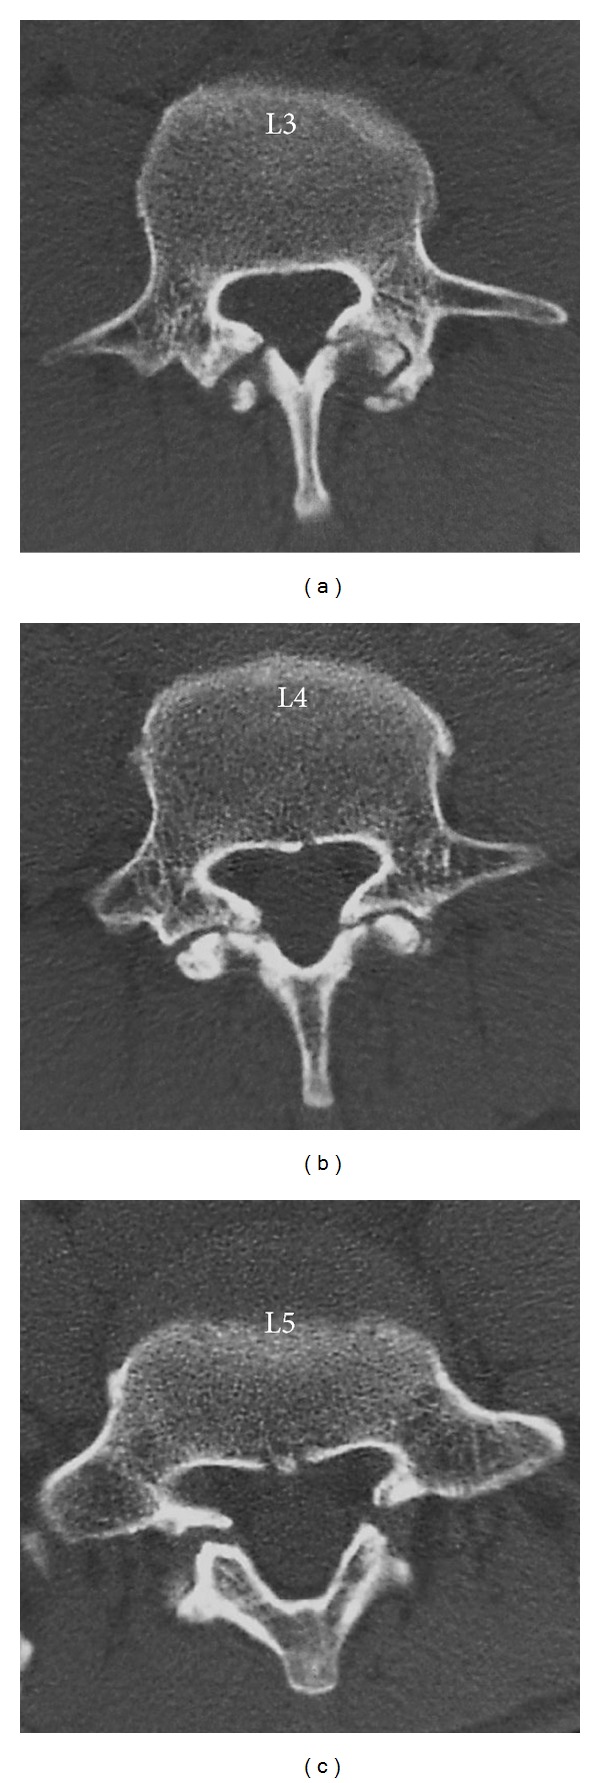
CT at initial presentation shows pseudarthrosis-type pars defects bilaterally at L3–5.
